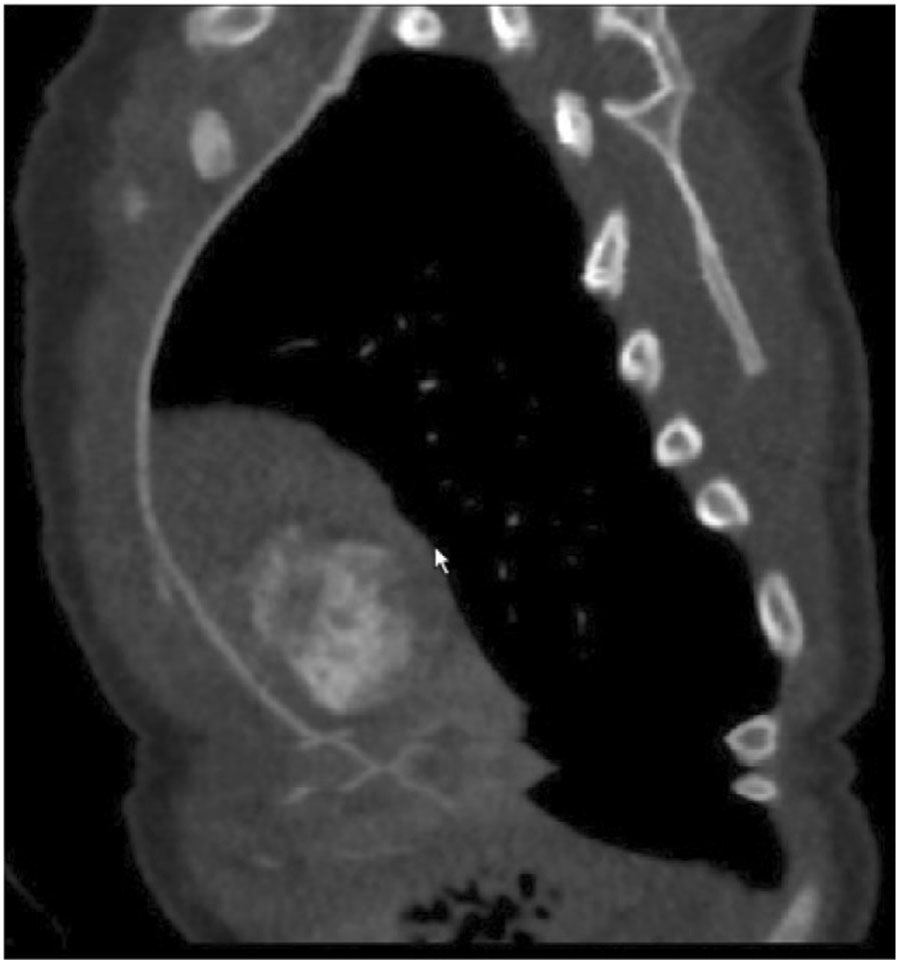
Pre-operative CT angiogram. Sagittal view obtained from pre-operative CT angiogram demonstrating left lower lobe sequestration with blood supply from the left internal thoracic artery
